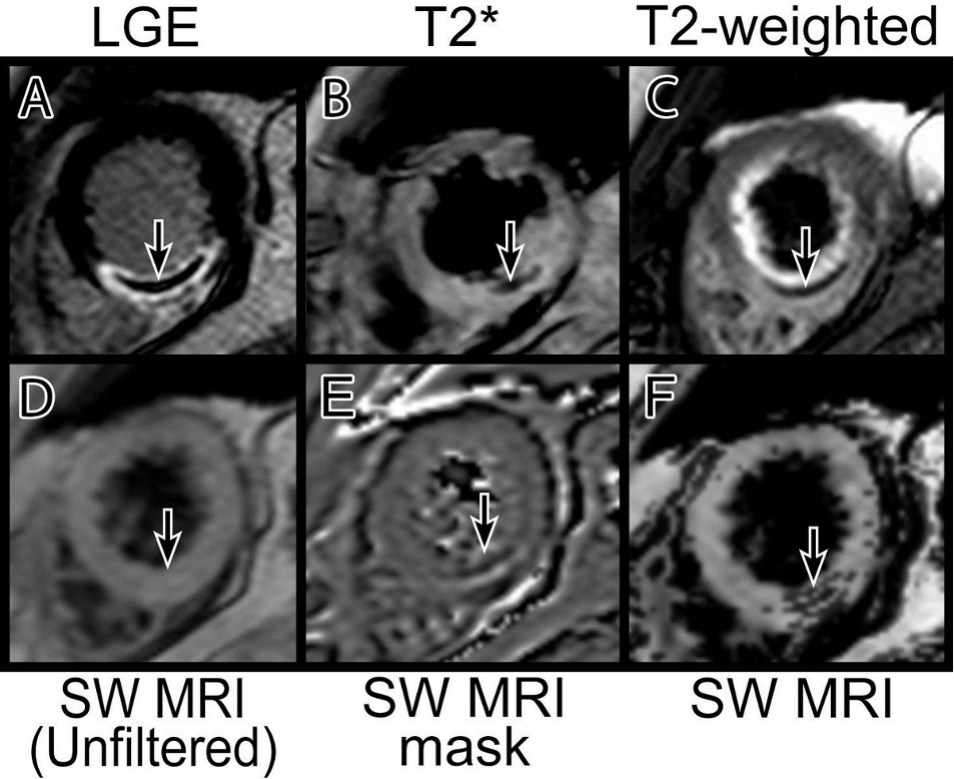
Inferior AMI with IMH (arrowed). Prior to phase enhancement, the SW MRI sequence has relatively low sensitivity for IMH (D). Application of the phase mask (E) results in clear visualization of IMH (F).
